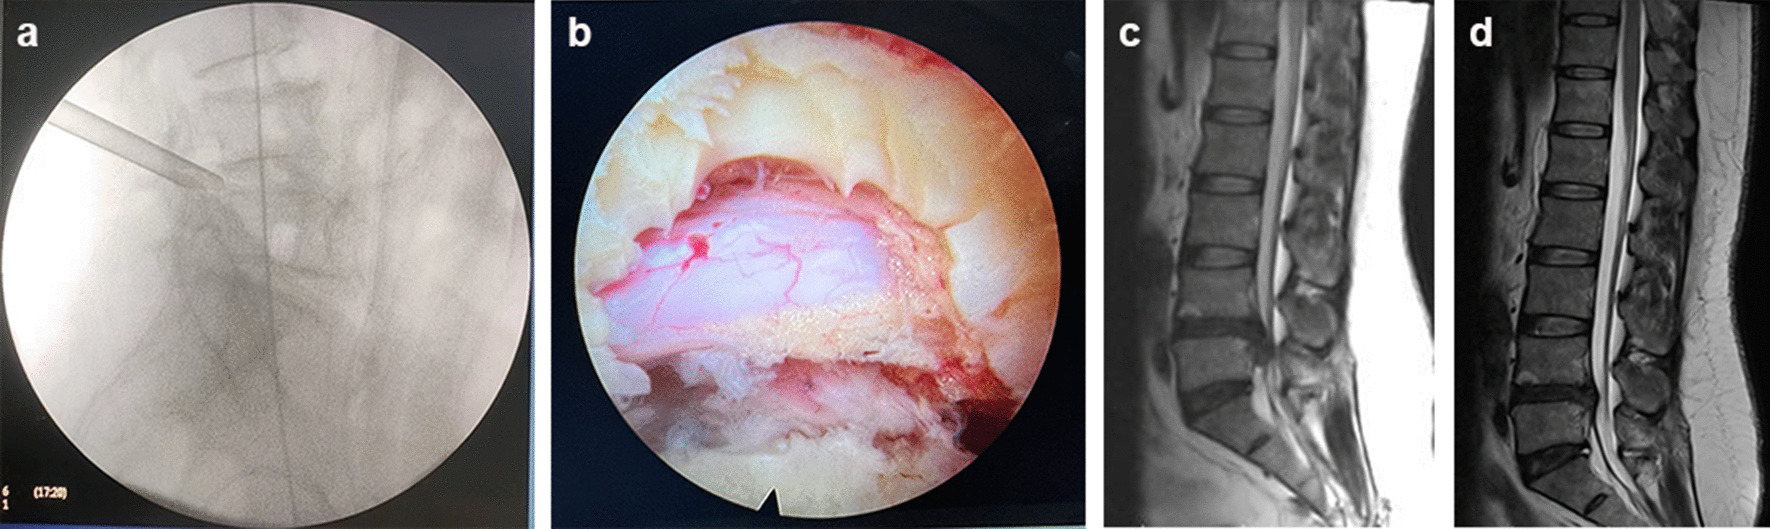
ETLD. a Preoperative fluoroscopy localization, b macroscopic observation of nerve roots under endoscope after operation, c preoperative MRI sagittal image, d post-operative MRI sagittal image
